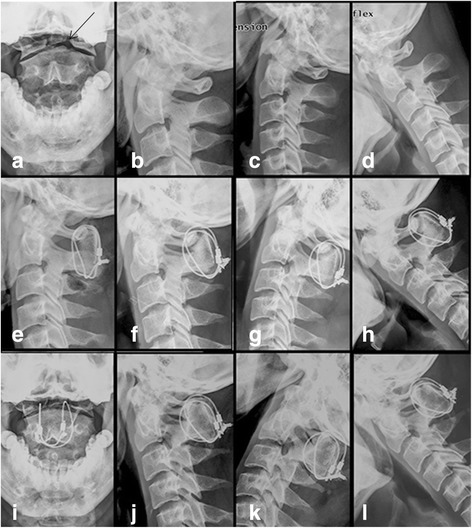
A 27-year-old man had old odontoid fracture caused by accident. a–d Preoperative X-ray showed fracture line was located at the bottom of odontoid (*arrow*), revealing an atlantoaxial instability. e–l X-ray obtained on the 1st day, the 3rd month, the 6th month, the 1st year, and the 2nd year postoperatively showed bone fusion
